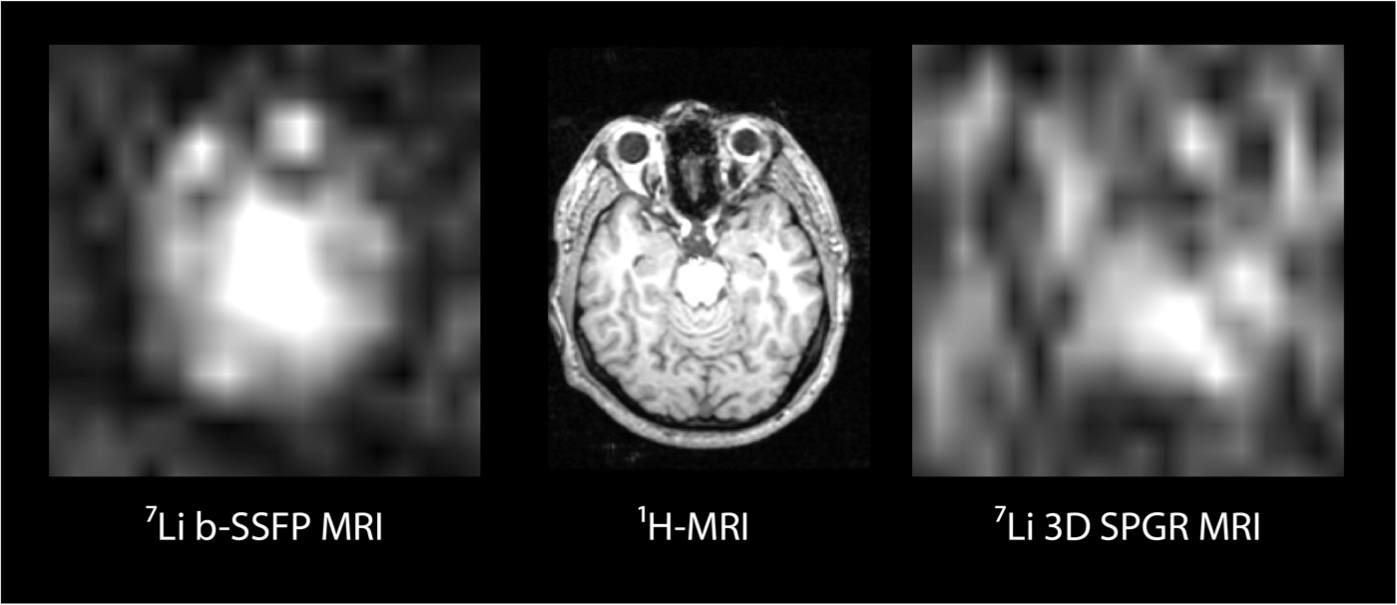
Comparison of the ^7^Li b-SSFP MRI and ^7^Li SPGR MRI in a single subject, plotted with the same intensity scale and acquired with equal scan durations (24 min in total for each sequence)
